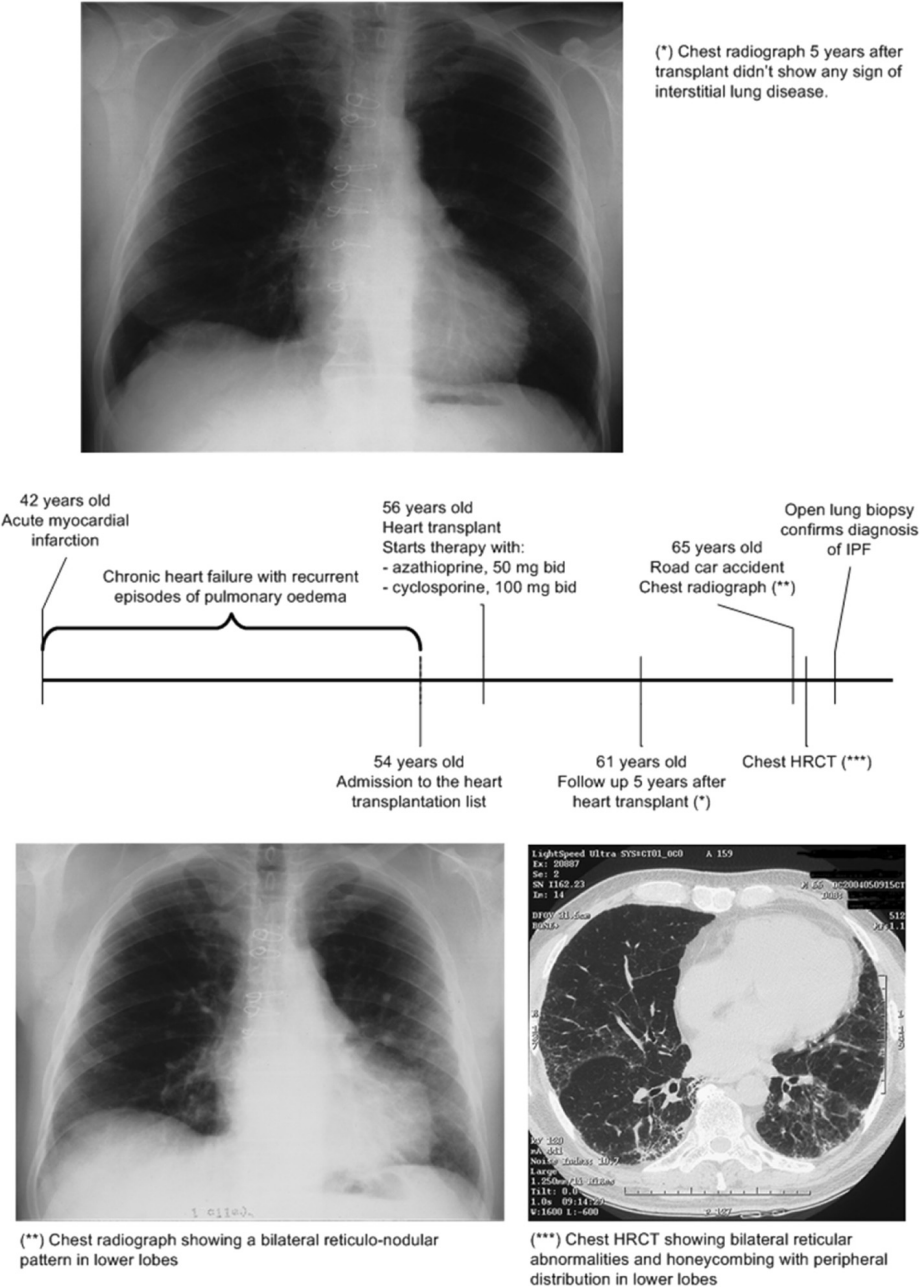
Timeline of the patient’s clinical history. *HRCT* high-resolution computed tomography, *IPF* idiopathic pulmonary fibrosis
